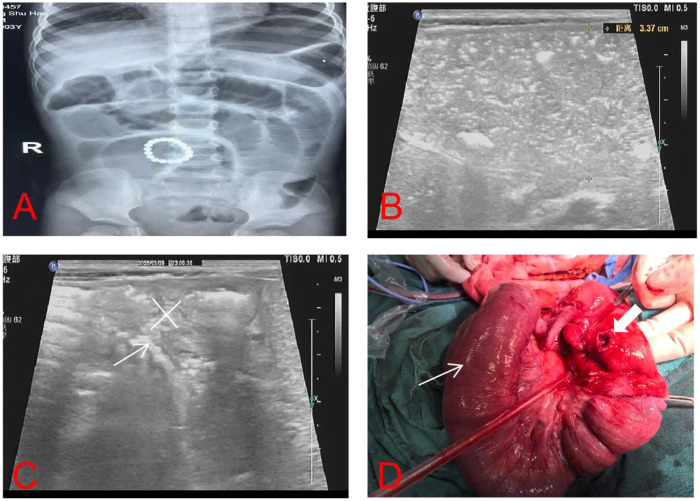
Imaging findings and intraoperative views of intestinal obstruction, internal hernia, and bowel perforation caused by magnetic beads (male child, 3 years old). (A) Abdominal x-ray shows partial dilation of intestinal loops in the upper abdomen with air–fluid levels; ring-shaped high-density shadows are seen in the mid–lower abdomen. (B) Abdominal ultrasound shows partial dilation of intestinal loops. (C) Abdominal ultrasound shows multiple hyperechoic foci with acoustic shadowing (arrows) in the abdominal cavity, along with a “cross-link adhesion” sign. (D) Intraoperative findings reveal an internal hernia; part of the intestine is dilated (thin arrow), and bowel perforation is visible (thick arrow).
